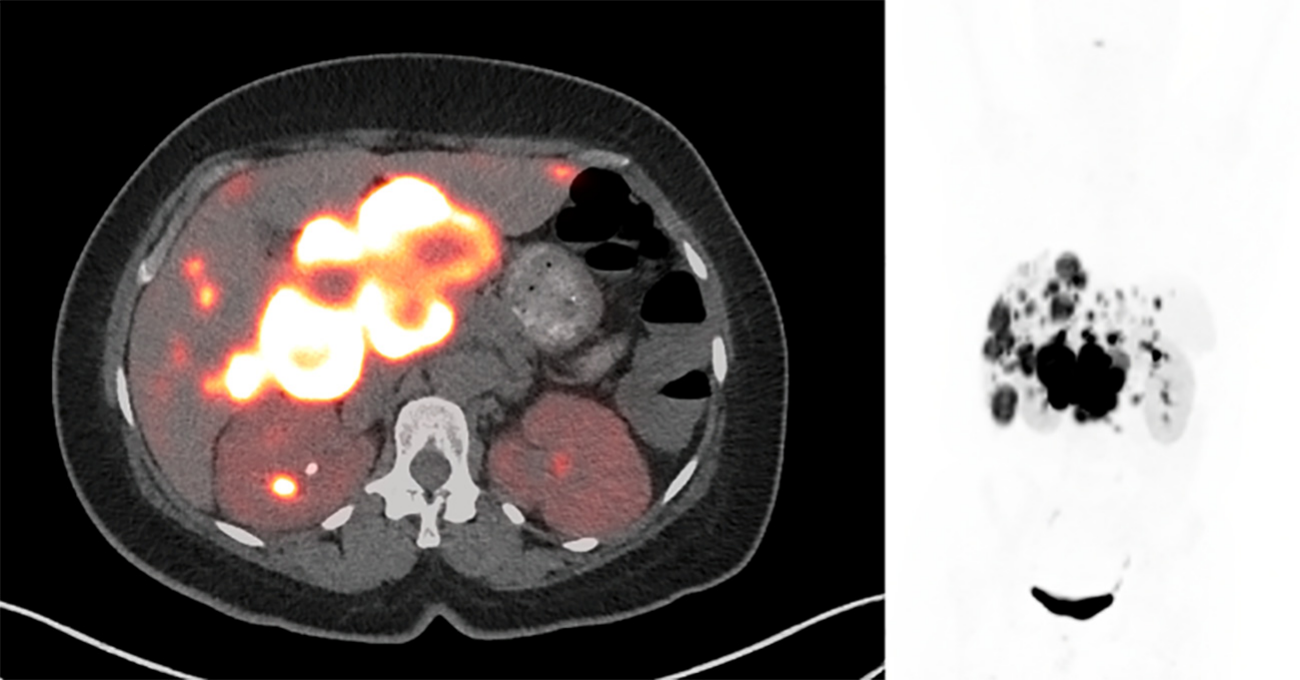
Post-chemotherapy ^68^Ga-DOTATATE PET/CT shows intense radiotracer uptake in the peripancreatic mass and liver lesions, which was not significantly changed compared to pre-treatment PET/CT. SUVmax of the peripancreatic mass was 82.4. There was interval resolution of the prominent activity in the right urinary collecting system.
